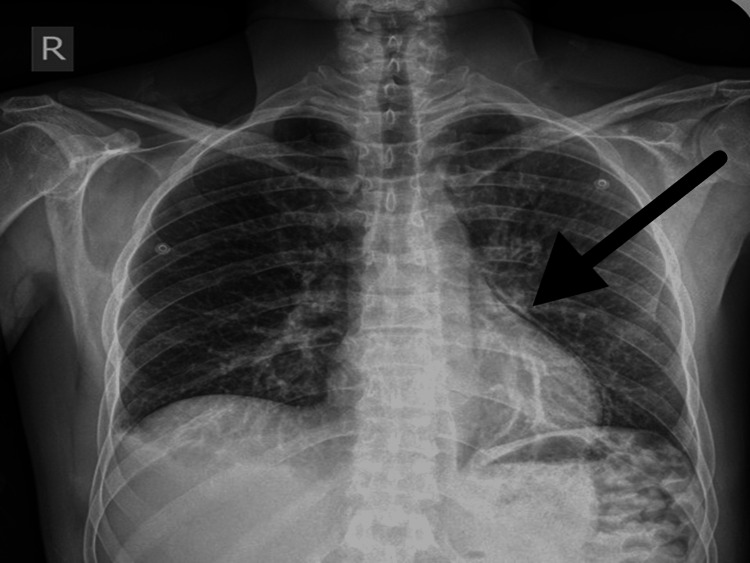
Admission x-ray demonstrating air in the pericardial sac.
